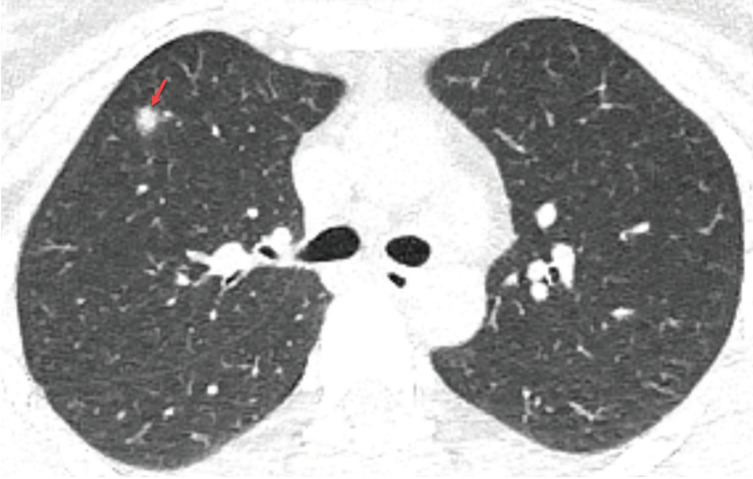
A 36-year-old woman presented with complaints of cough and fever for 2 days. Axial chest CT image shows a nodule with halo sign in the right upper lobe (red arrow). The nasopharyngeal swab test of the patient was positive for 2019-nCoV, and the nodule disappeared at follow-up.
